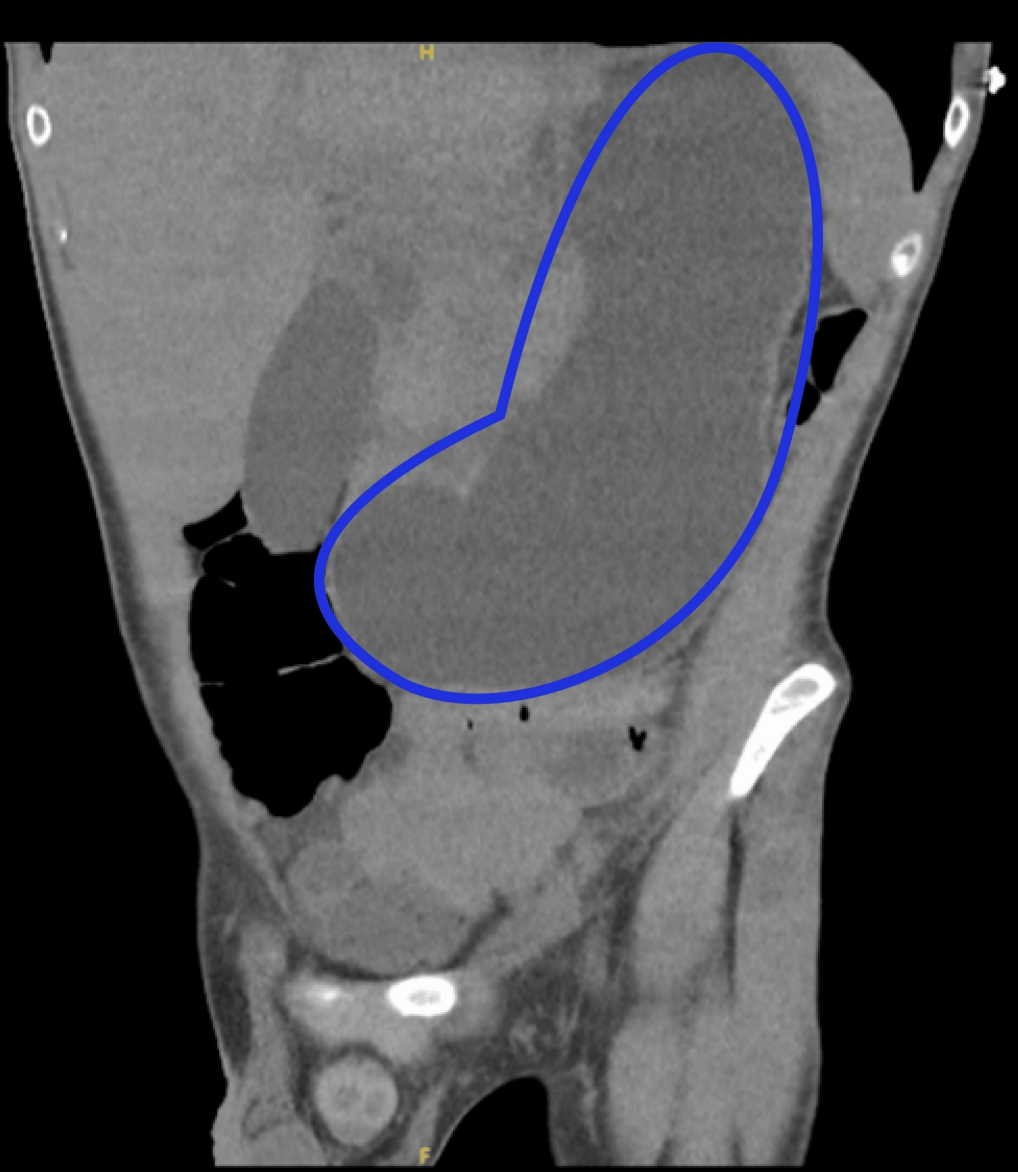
CT abdomen showing profound gastric dilatation
